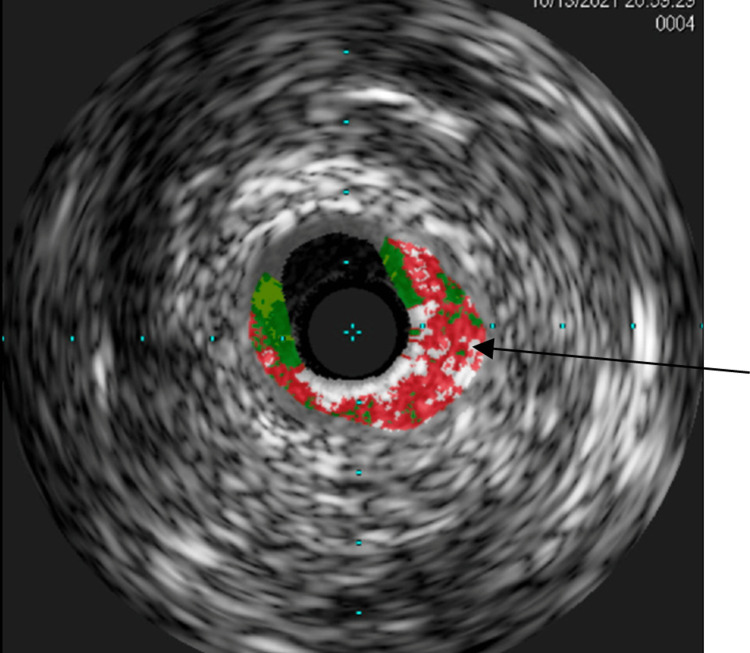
Dissection shown with IVUS imaging of the LAD. The red blood cells extravasating outside of the lumen of the vessel are represented with the black arrow. IVUS, intravascular ultrasound; LAD, left anterior descending
